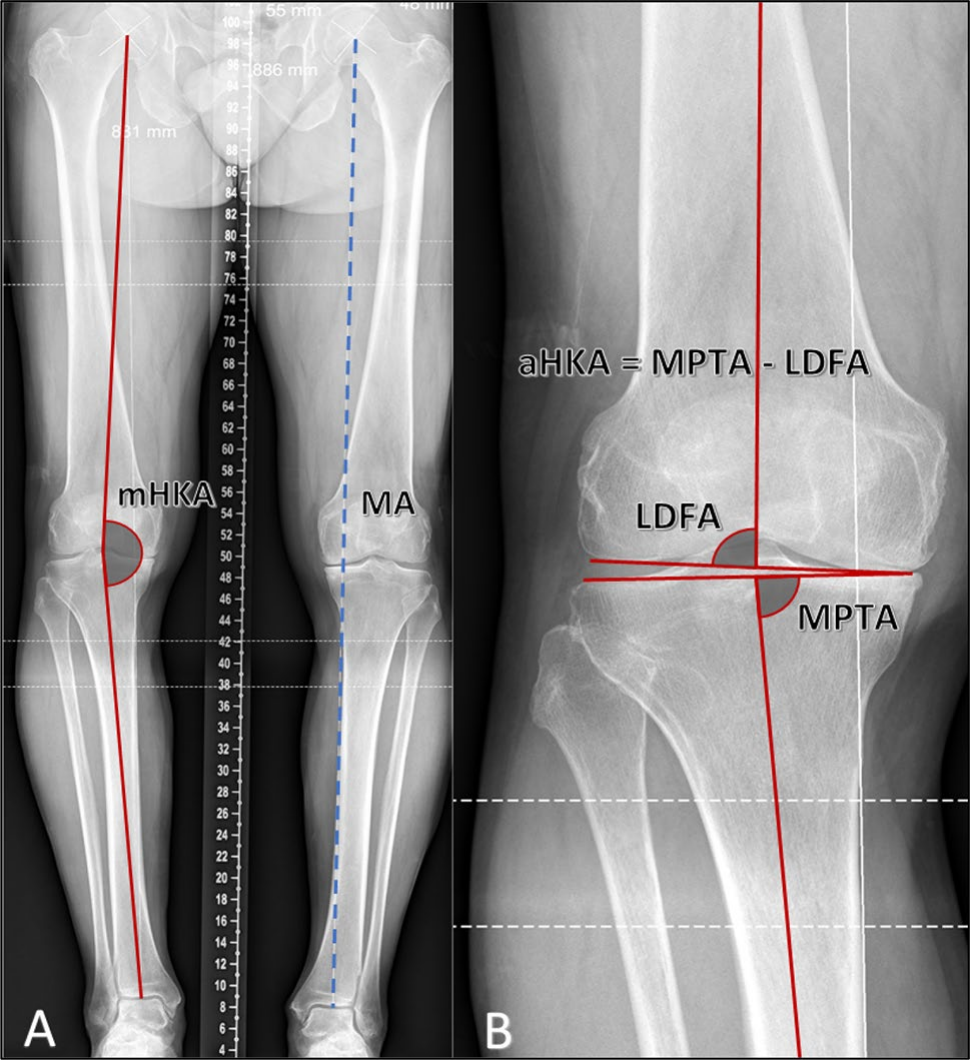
Determination of the arithmetic HKA angle on radiographs. A Full preoperative long leg standing radiograph. In the right limb, the mechanical HKA (mHKA) is highlighted. The mHKA is the angle subtended by the MA femur and the MA tibia. The MA of the lower limb, shown on the left leg, is the line marked from the centre of the hip joint to the centre of the ankle joint. B Calculation of constitutional alignment in the arthritic knee using the aHKA algorithm, which subtracts the lateral distal femoral angle (LDFA) from the medial proximal tibial angle (MPTA). *mHKA* mechanical hip-knee-ankle angle, *MA* mechanical axis, *aHKA* arithmetic hip-knee-ankle angle, *LDFA* lateral distal femoral angle, *MPTA* medial proximal tibial angle
